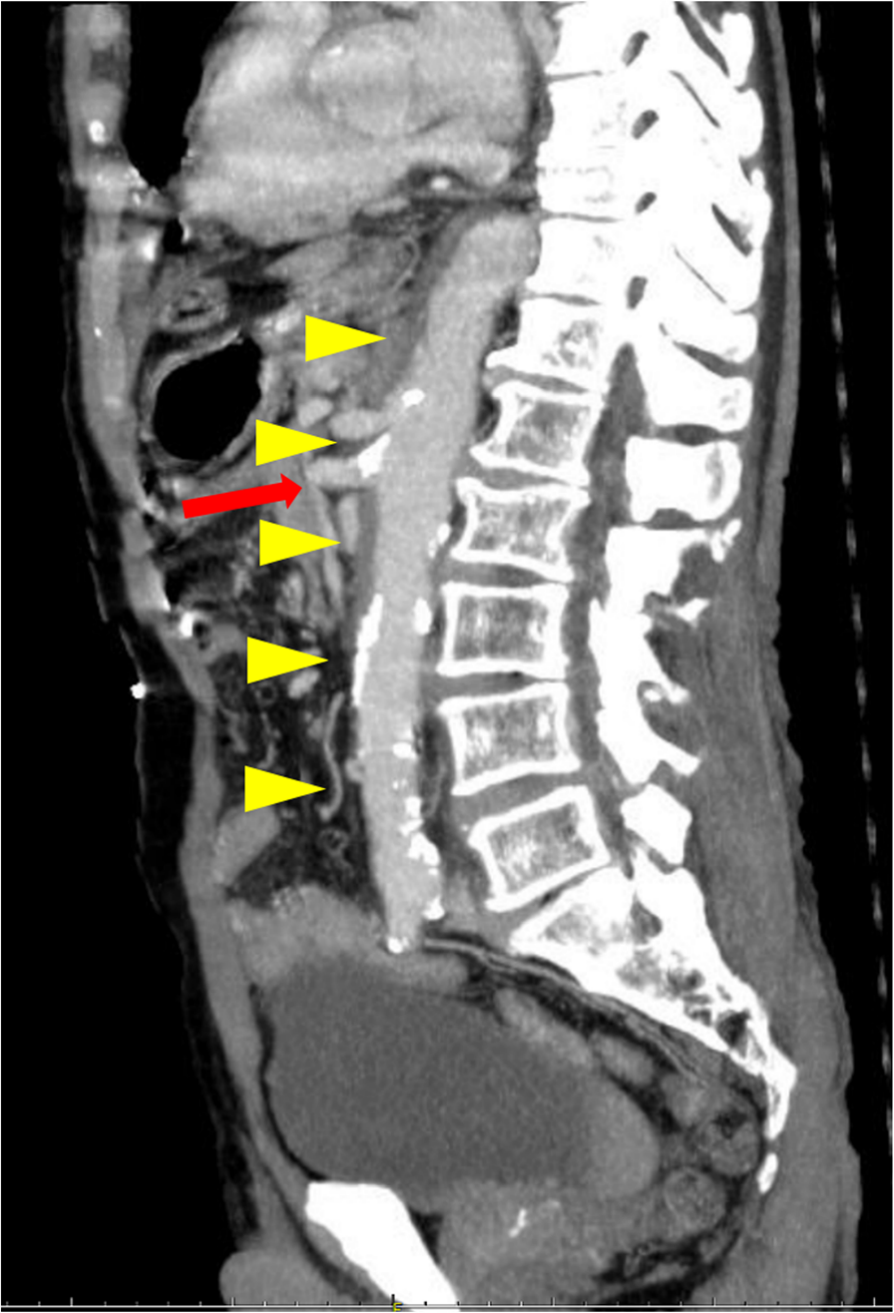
Contrast-enhanced CT indicated a type B AD (yellow arrowheads) including the SMA (red arrow)
